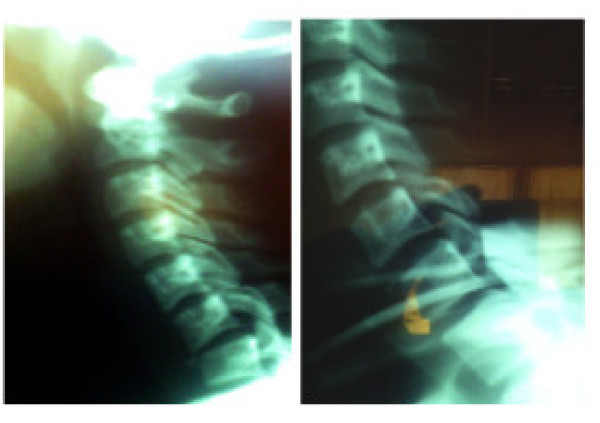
Lateral pre and post reduction x-rays.
